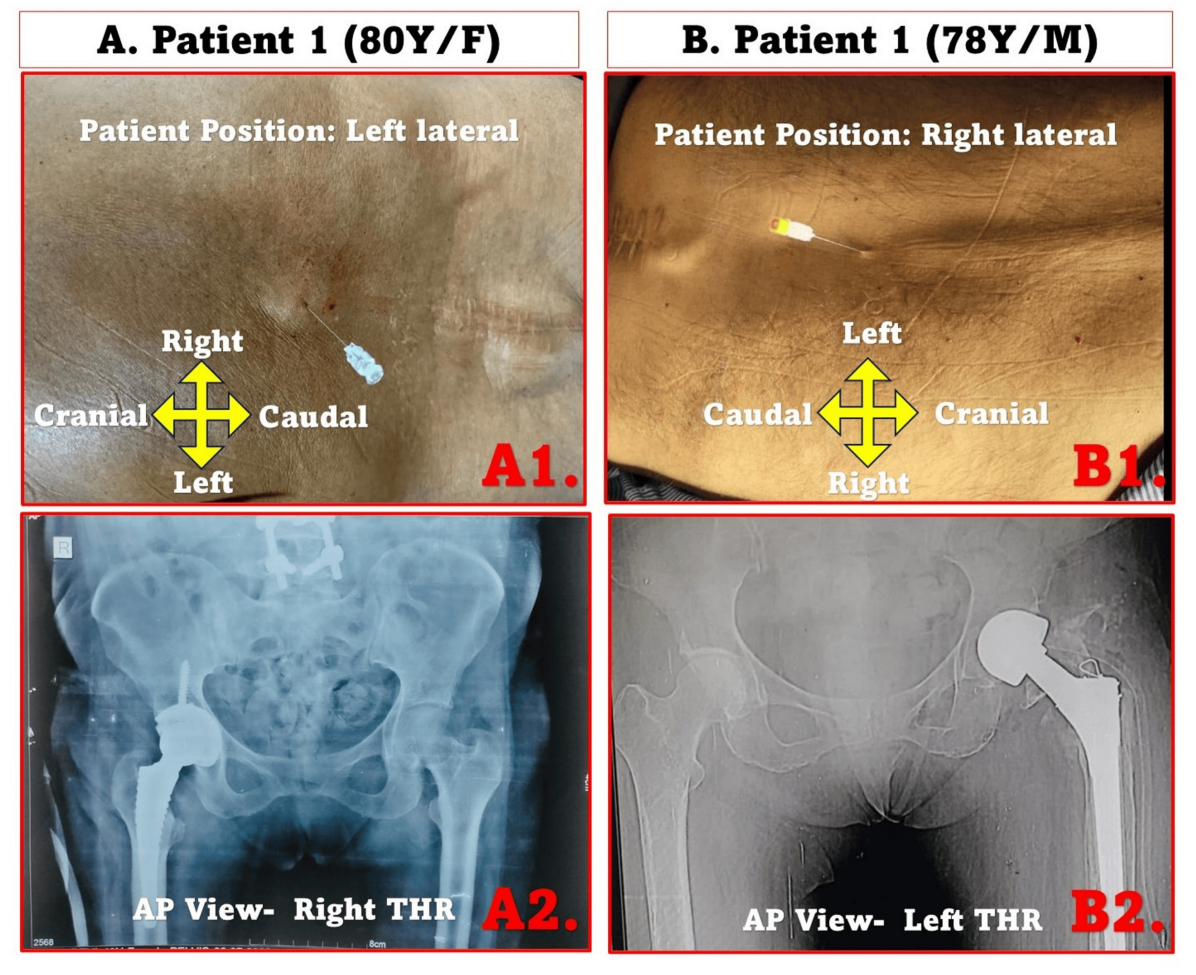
Clinical and radiological findings of two geriatric patients undergoing thoracic spinal anesthesia for total hip replacement. (A1) Spinal puncture performed above the lumbar scar in an 80-year-old female, left lateral position. (A2) Anteroposterior (AP) pelvic radiograph showing right total hip replacement (THR). (B1) Spinal puncture in a 78-year-old male, right lateral position. (B2) AP pelvic radiograph showing left THR. AP: anteroposterior; THR: total hip replacement.
